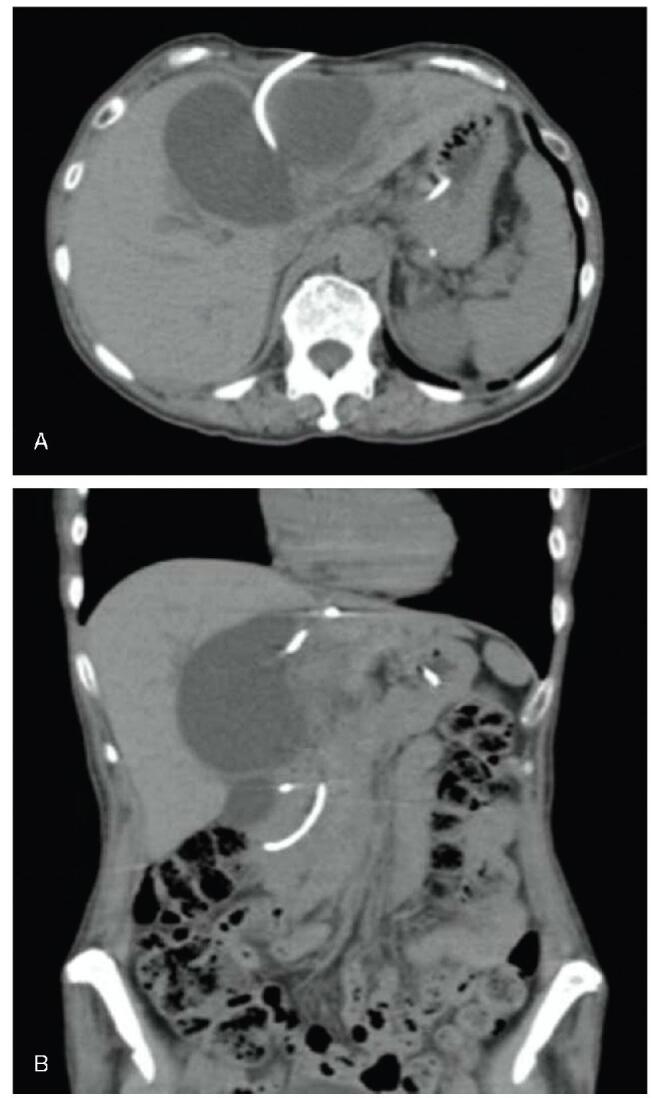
Figure 4. Abdominal computed tomography (CT) showing enlargement of the liver cyst. After the endoscopic nasobiliary drainage tube was placed in the common bile duct, and the percutaneous drainage tube was clamped, abdominal CT was performed, which showed that the liver cyst increased in size.
